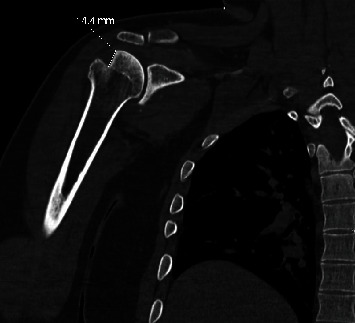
Coronal CT scan, right shoulder, postreduction (case 1). The large Hill-Sachs lesion of the humeral head is clearly seen, with nearly 15 mm of bony impaction.
